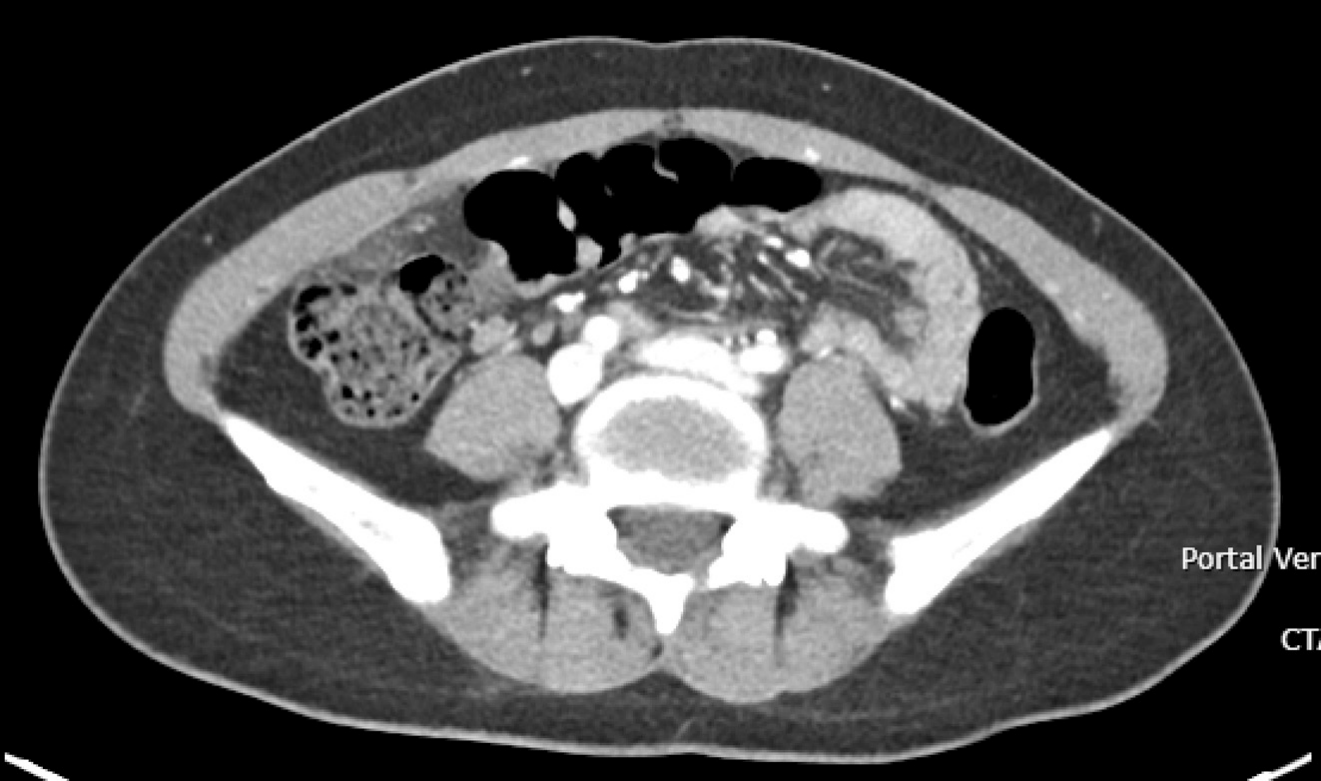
Axial View of CT abdomen/pelvis demonstrated inflammatory fat stranding suggestive of omental infarction.
